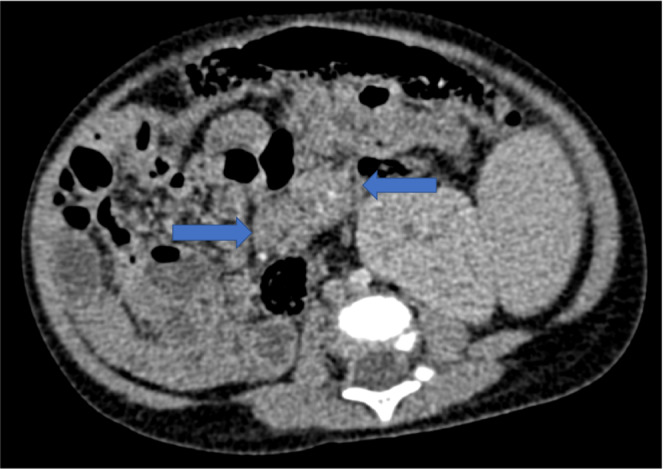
Enlarged retroperitoneal lymphadenopathies. Contrast‐enhanced computed tomography showed enlarged retroperitoneal lymphadenopathies (arrows) that were considered for excisional biopsy, but a biopsy was not performed due to the relative inaccessibility of the lesions.
